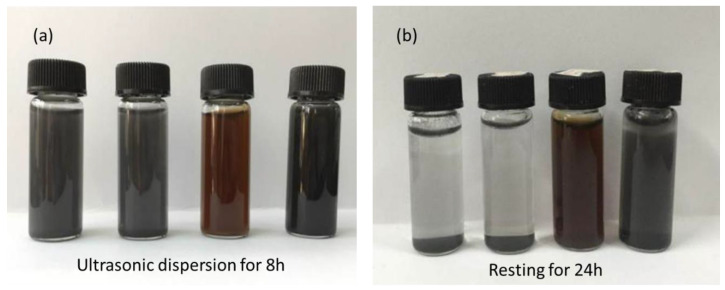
Dispersion of graphite, oxidized expanded graphite, graphene oxide, and active graphene dispersion, from left to right respectively, in: (a) Ultrasonic dispersion for 8 h; (b) resting for 24 h.
